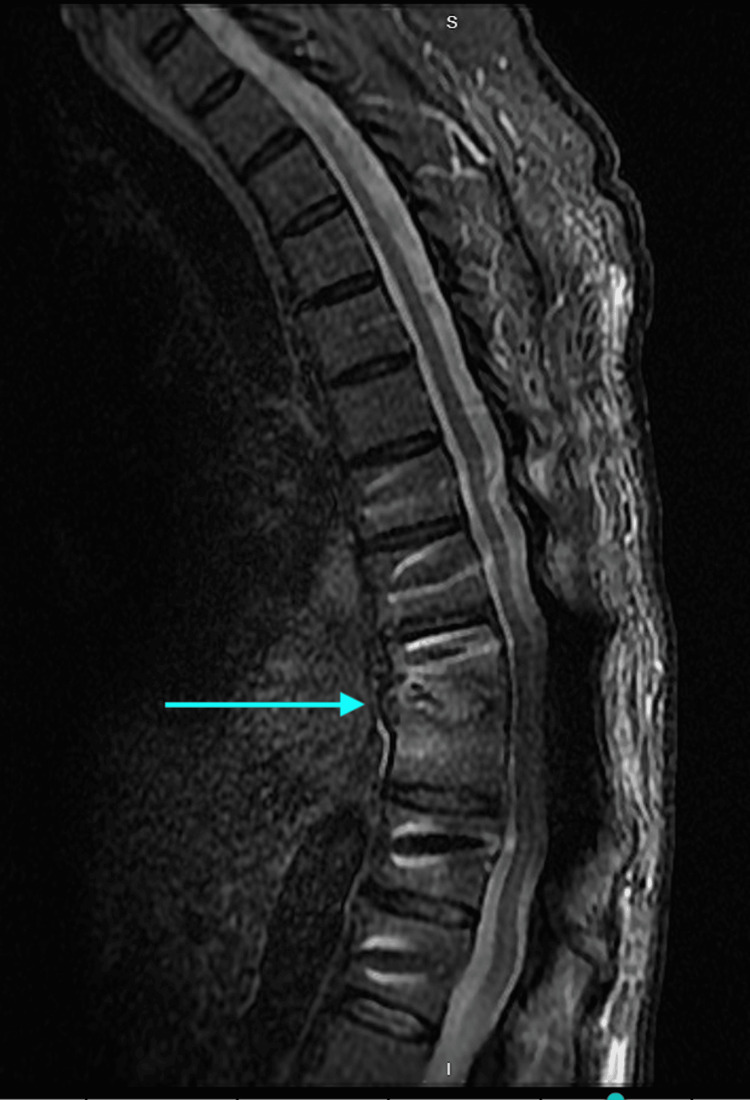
MRI of the thoracic spine with the T9-T10 area now with significant improvement in the lesion.
